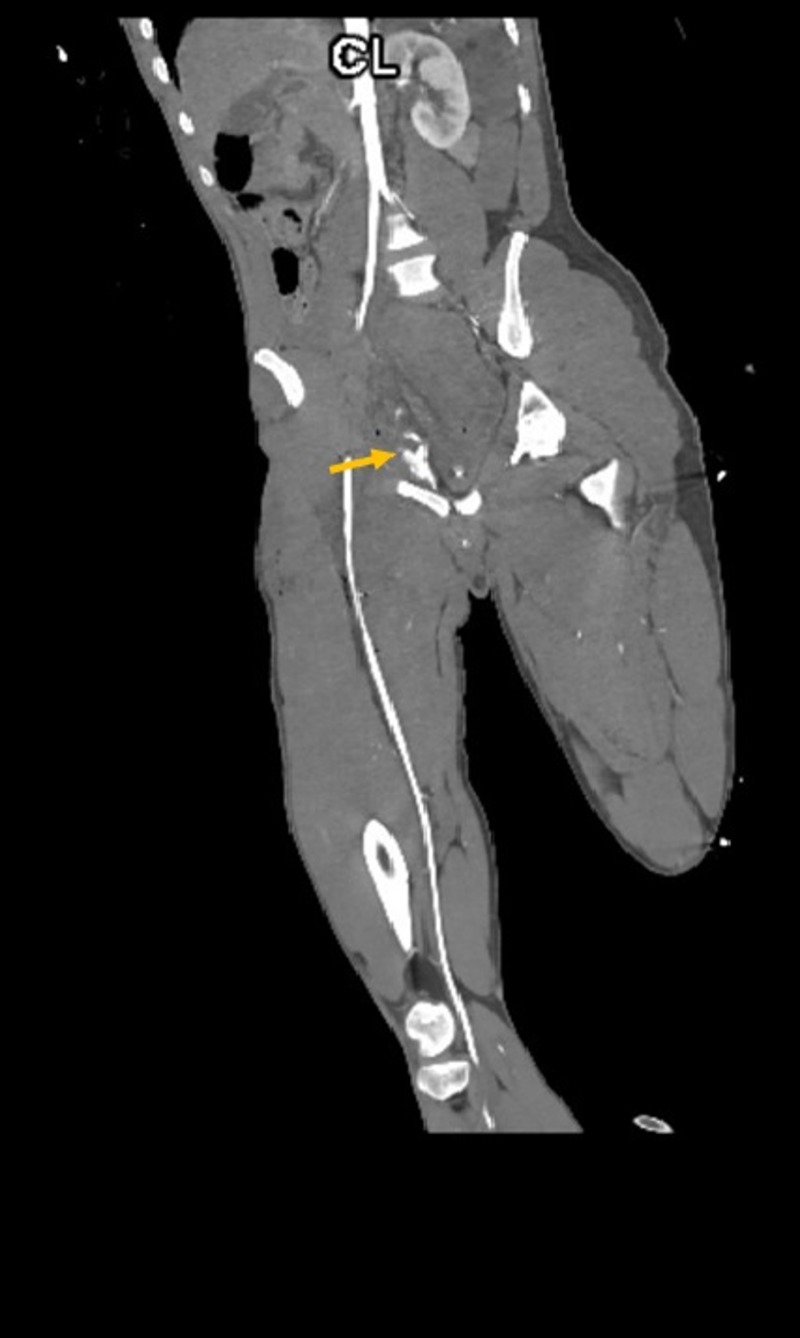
Computed tomography angiography (CTA) of the pelvis (coronal view) Reconstructed coronal view of abdominal aorta and bilateral lower extremities demonstrating blush (arrow) at the superior pubic ramus, consistent with right obturator artery injury.
